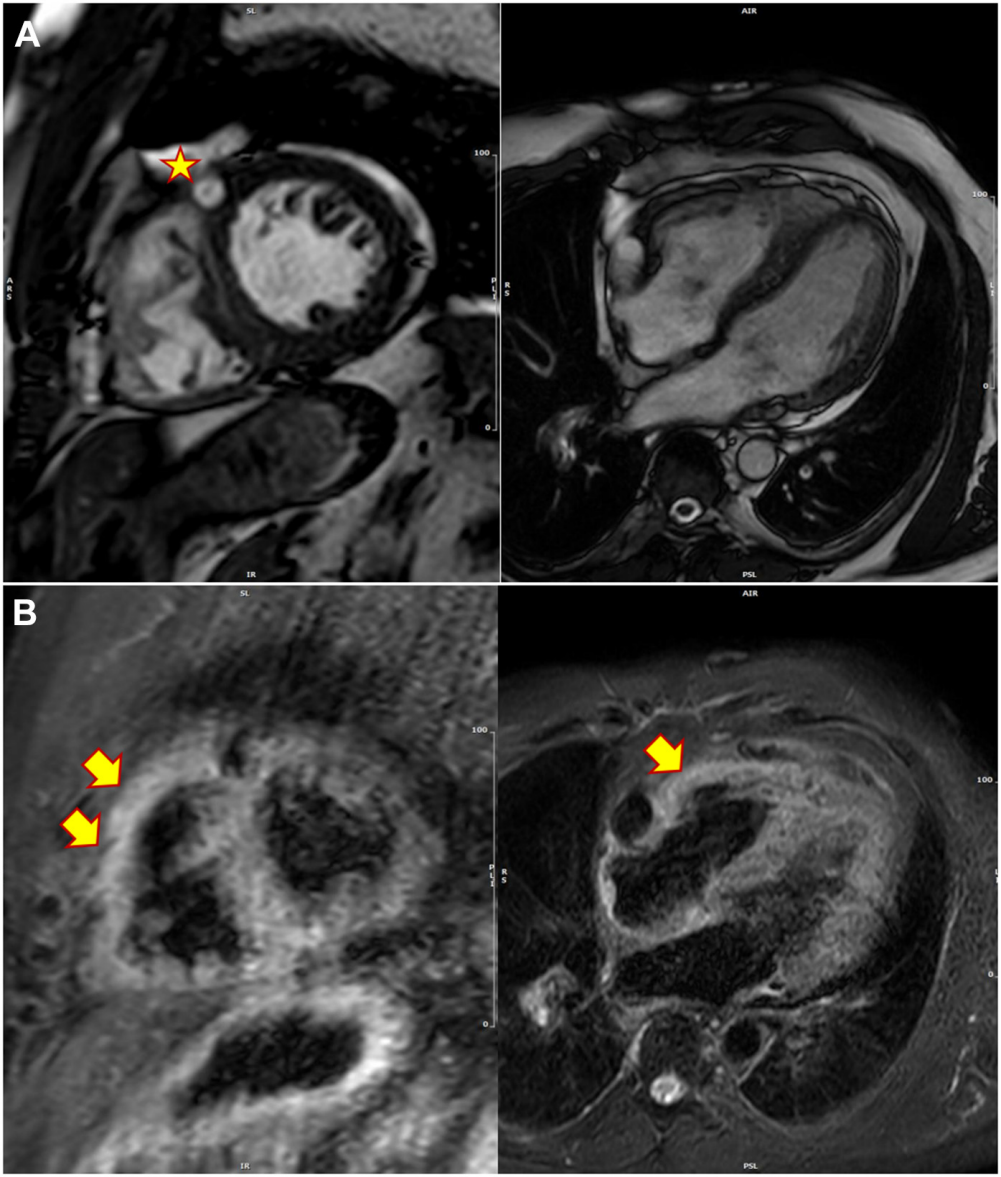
Cardiac MRI cine (A) and T2 STIR (B) sequences demonstrating the presence of a dilated left anterior descending artery (star), with subtle evidence of myocardial oedema in the right ventricular free wall (arrows), likely because of pulmonary embolism.
